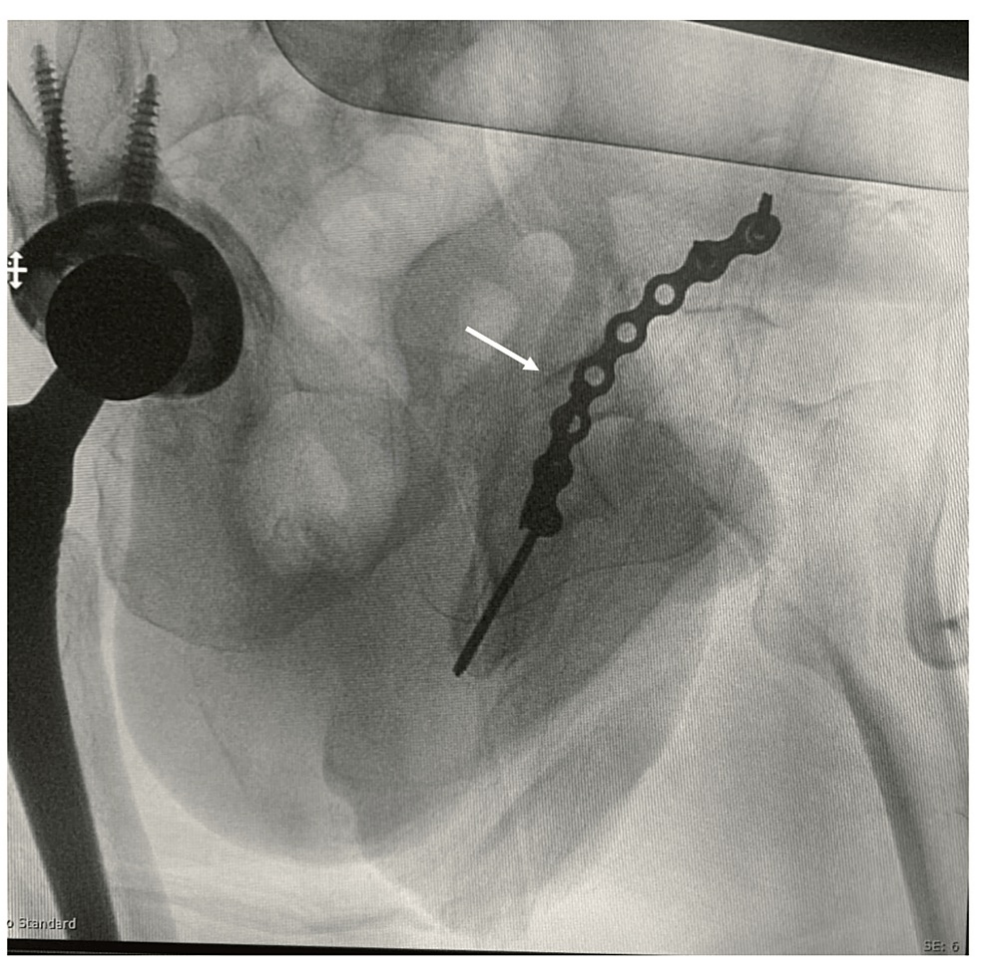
Intra-operative iliac oblique view x-ray showing fixation of the posterior column with plate and screws.
